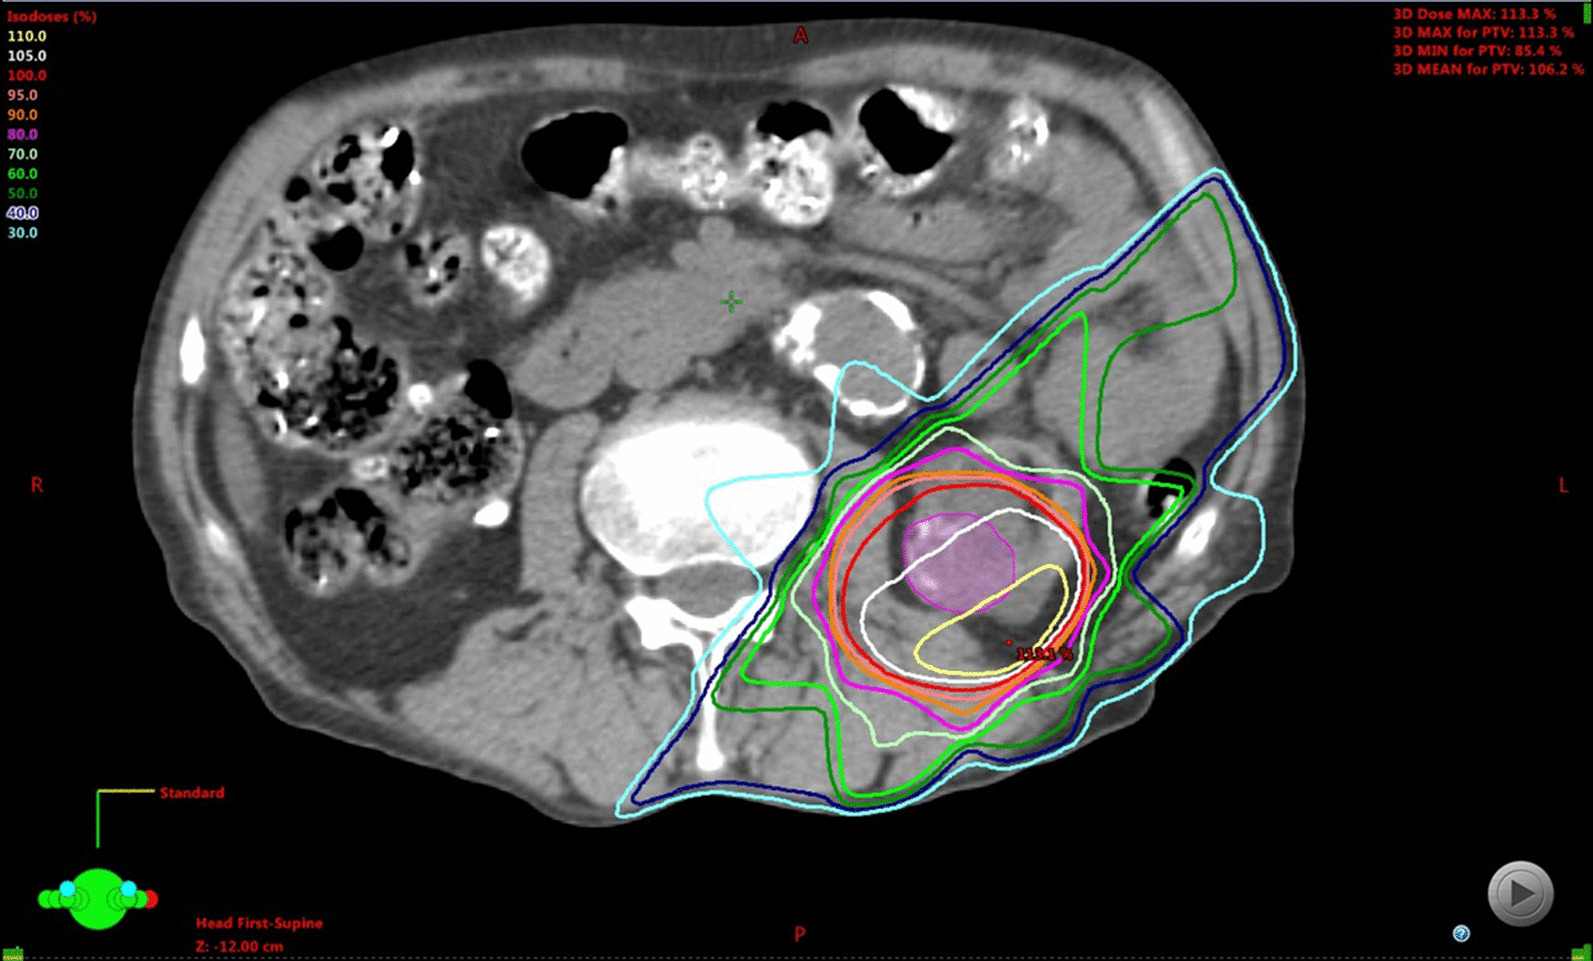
Axial image of dose distribution of stereotactic body radiotherapy (SBRT). Stereotactic body radiotherapy was performed with seven non-coplanar static 6 MV X-ray beams to the lesion of the right kidney using daily cone-beam computed tomography scans before each fraction. The patient was prescribed 70 Gy/10 fractions for the isocenter.
